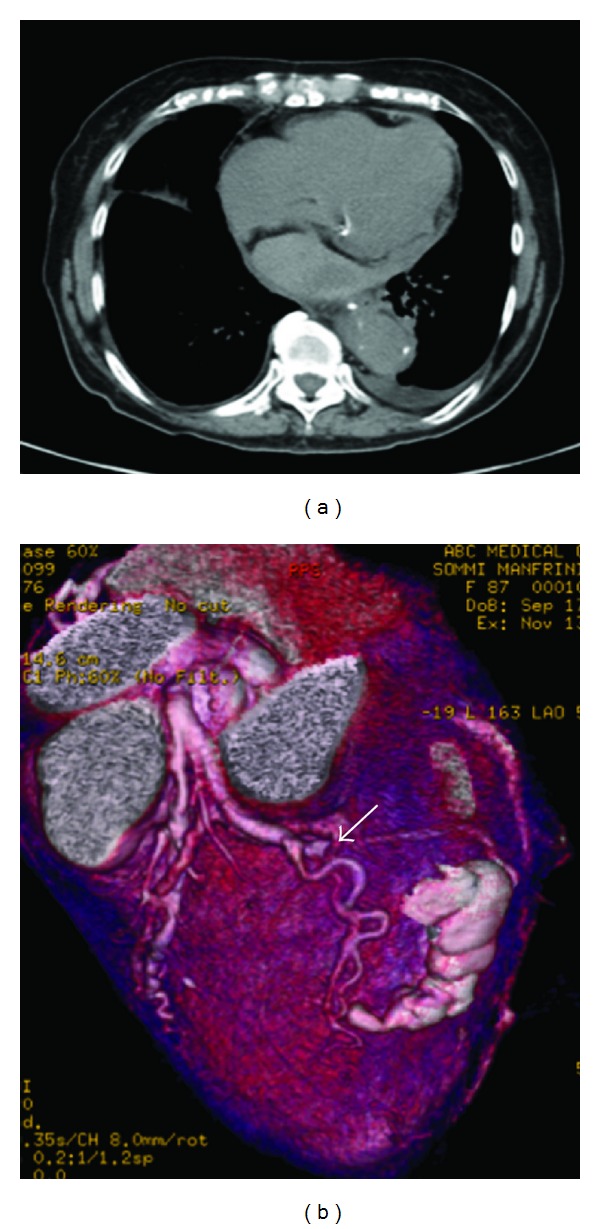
In (a) an important thickening of the pericardium containing the hemopericardium is seen and in (b) the cardiac hybrid CTA image shows a significative obstruction of circumflex artery in its middle segment (white arrow).
